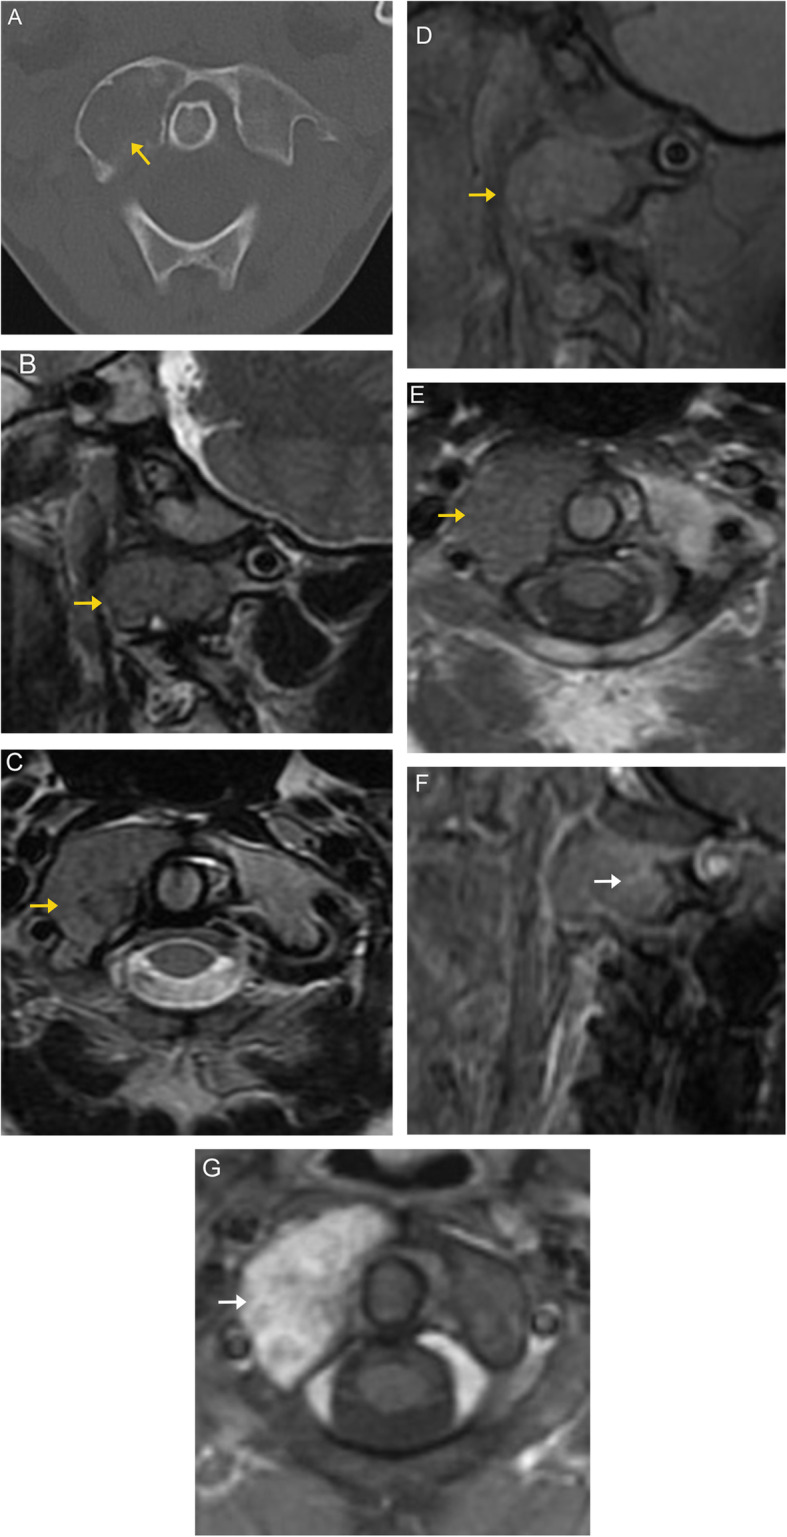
Osteosarcoma. Axial bone window CT image (a) demonstrates moth-eaten destruction of the right lateral mass of C1 (arrow), with a wide zone of transition along the posteromedial margin. Sagittal (b) and axial (c) T2-weighted images demonstrate the lesion to be low to intermediate in signal (arrow). The lesion is also low signal on T1-weighted images (d, e), and demonstrates avid enhancement (arrows) on post-contrast T1 sequences (f, g)
